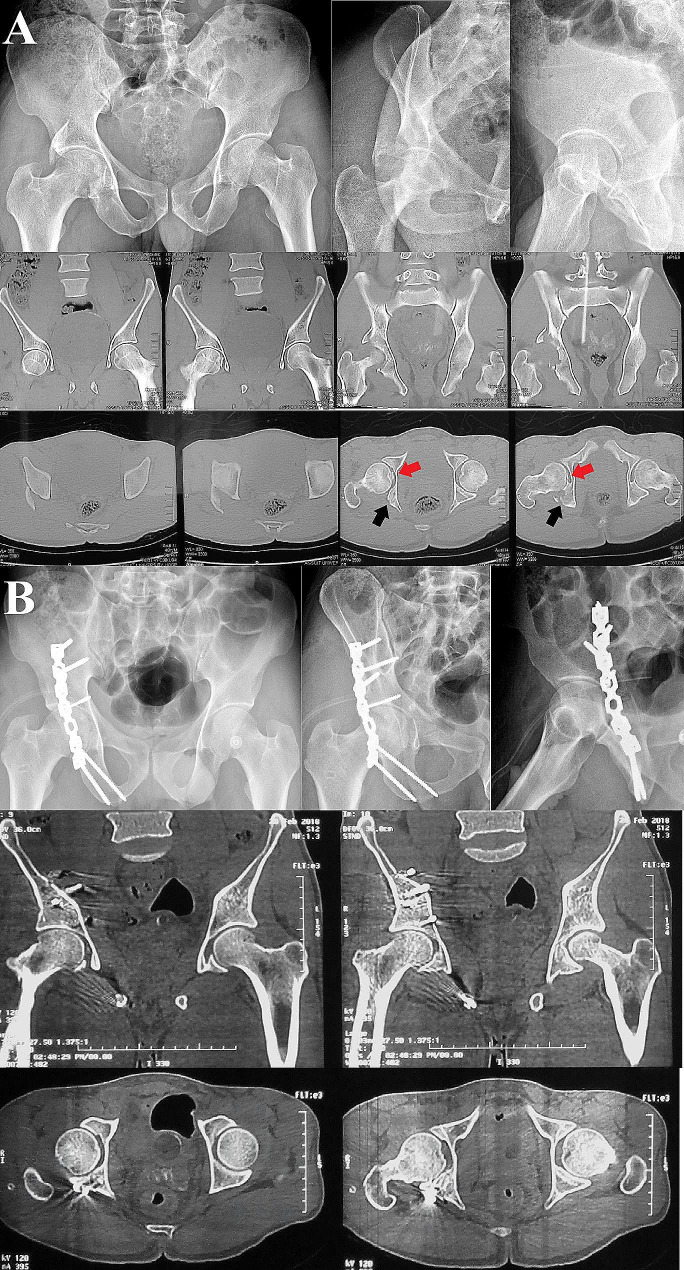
A male patient, 31 years old, presented with a posterior wall fracture of the right acetabulum associated with marginal impaction. A, preoperative plain radiographs and CT scans showing fracture of the posterior wall, marginal impaction injury (black arrow), and retained intraarticular loose fragment (red arrow). B, post-operative plain radiographs and CT scans showing fracture anatomical reduction and clearance of the hip joint space
